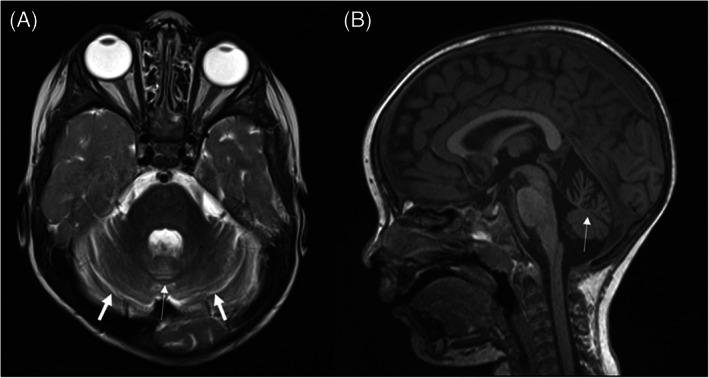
MRI brain performed at 2 years old showing cerebellar atrophy affecting the vermis (thin arrow) more than the cerebellar hemispheres (thick arrows)
